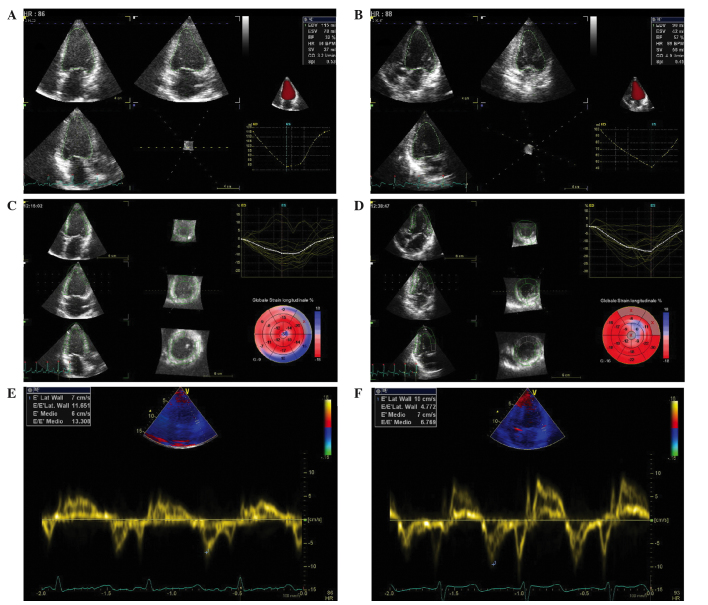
Depression of cardiac function during severe hypocalcemia (3.7 mg/dl) and restoral following serum calcium normalization. (A) 3D echocardiography indicated a 3D-LVEF of 32% (normal, >55%) with left ventricular dilatation. (B) Volume normalization and 3D-LVEF of 57% following treatment. (C) GLS reduction at speckle tracking (−9%). (D) Recovery following calcium normalization (GLS, −16%). (E) Abnormal E/E' ratio (13.3, normal values <8; non-invasive reliable estimation of the degree of ventricular filling pressures) detected by 3D-tissue Doppler. (F) Normalization following calcium restoration (E/E', 6.7). GLS, global longitudinal strain; 3D, 3-dimensional; LVEF, left ventricular ejection fraction.
